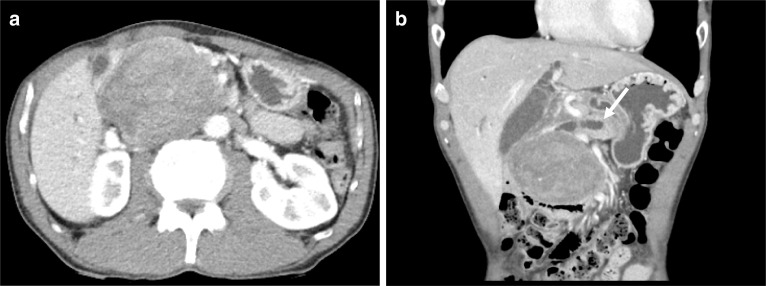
Abdominal CT. Enhanced CT shows a 7 × 9 × 7 cm tumor located in the pancreatic head (a). Dilatation of the distal pancreatic duct is seen (arrow) (b)
